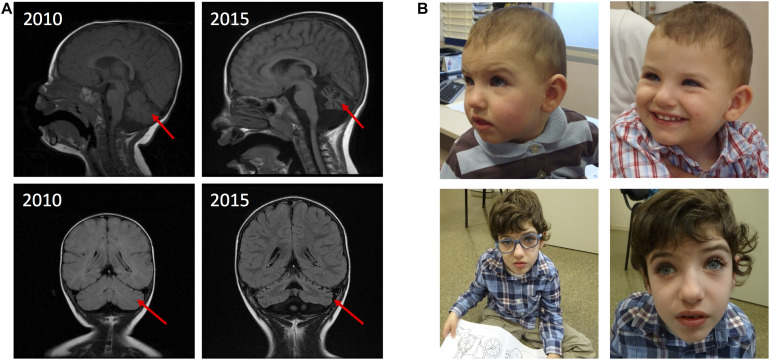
MRI findings and pictures of the patient. (A) MRI showing progressive cerebellar atrophy. (B) Patient at 1 year of age (top left), at 1 year and 9 months of age (top right) and at 8 years of age (in the bottom).
